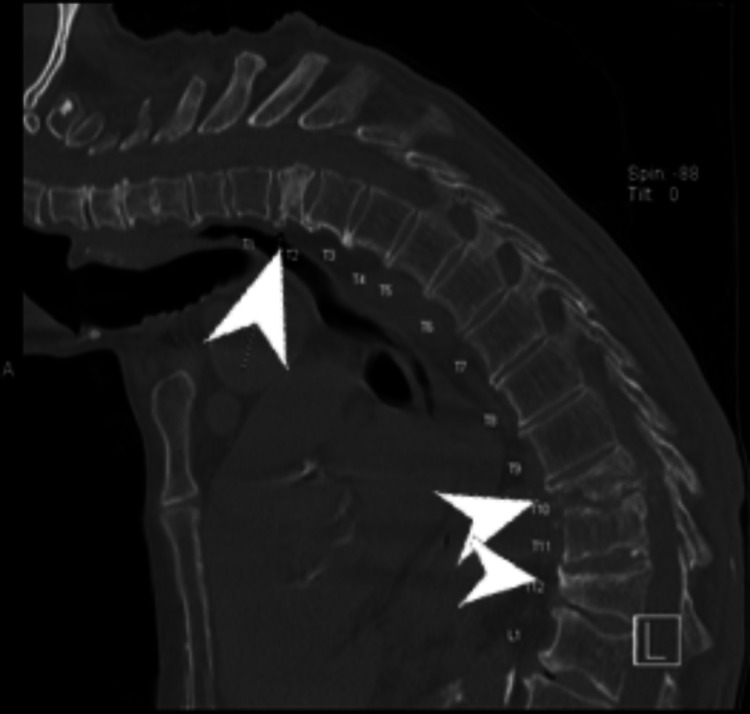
Repeat CT scan of the spine without contrast The image shows severe kyphosis of the thoracic spine. There is a compression fracture of the T2 vertebral body with sclerosis and this is mild compression deformity. There are also severe compression deformities of the T10 and T12 levels with extensive endplate changes in the region CT: computed tomography
